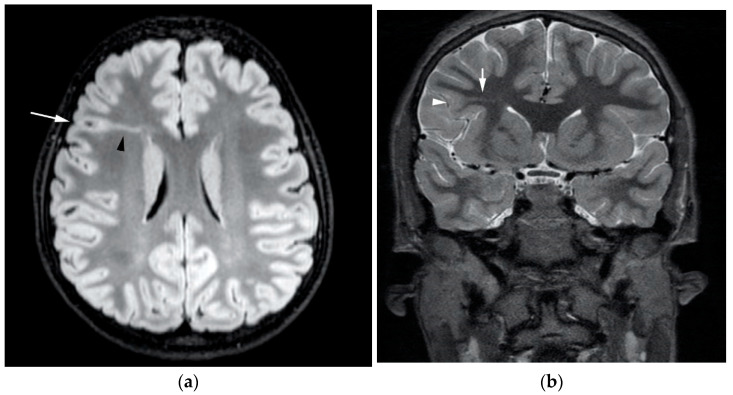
(a) Axial FLAIR image of a focal cortical dysplasia type IIb in the right frontal cortex with subtle blurring of grey–white differentiation (white arrow) and transmantle sign (black arrowhead), extending to the anterior body of the right lateral ventricle. The transmantle sign is diagnostic of FCD IIb. (b) Coronal T2-weighted image showing the same right frontal FCD IIb (white arrowhead) with transmantle sign (white arrow) seen in (a).
